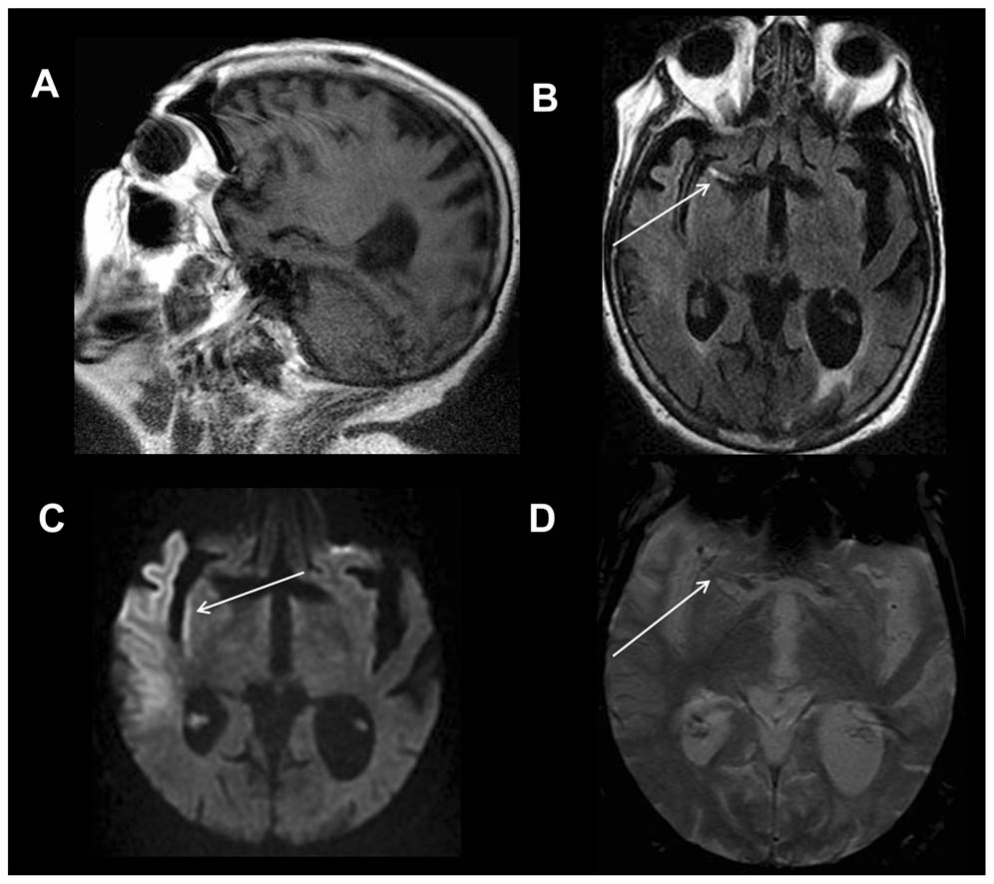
Acute stroke in a 78 year old female with altered mental status. Sagittal T1-weighted image of the right temporal lobe/insula (A) is normal. Axial T2-weighted FLAIR image (B) shows hyperintense signal within the M1 segment of the right middle cerebral artery (MCA), suggesting slow flow or clot (white arrow). Axial diffusion weighted image (DWI) (C) shows restricted diffusion within the right temporal lobe and insula, diagnostic of acute stroke to this region (white arrow). Axial gradient recalled echo (GRE) susceptibility image (D) shows subtle linear susceptibility effect within the right MCA, consistent with recent passage of embolic material (white arrow).
